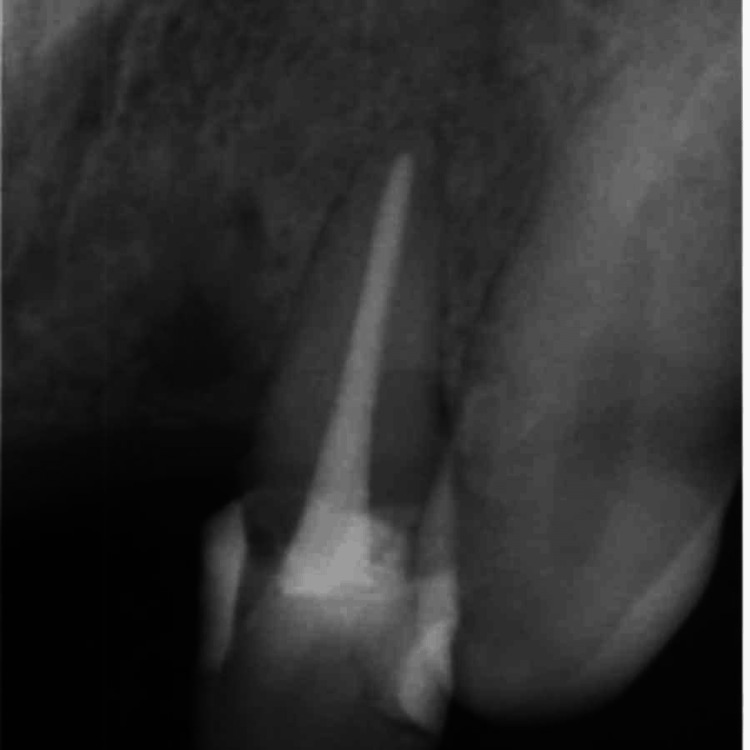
Postoperative intra-oral periapical radiograph
